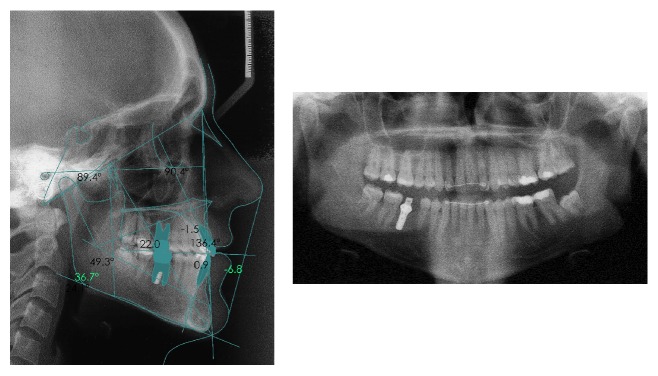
Lateral and panoramic final radiographies.
